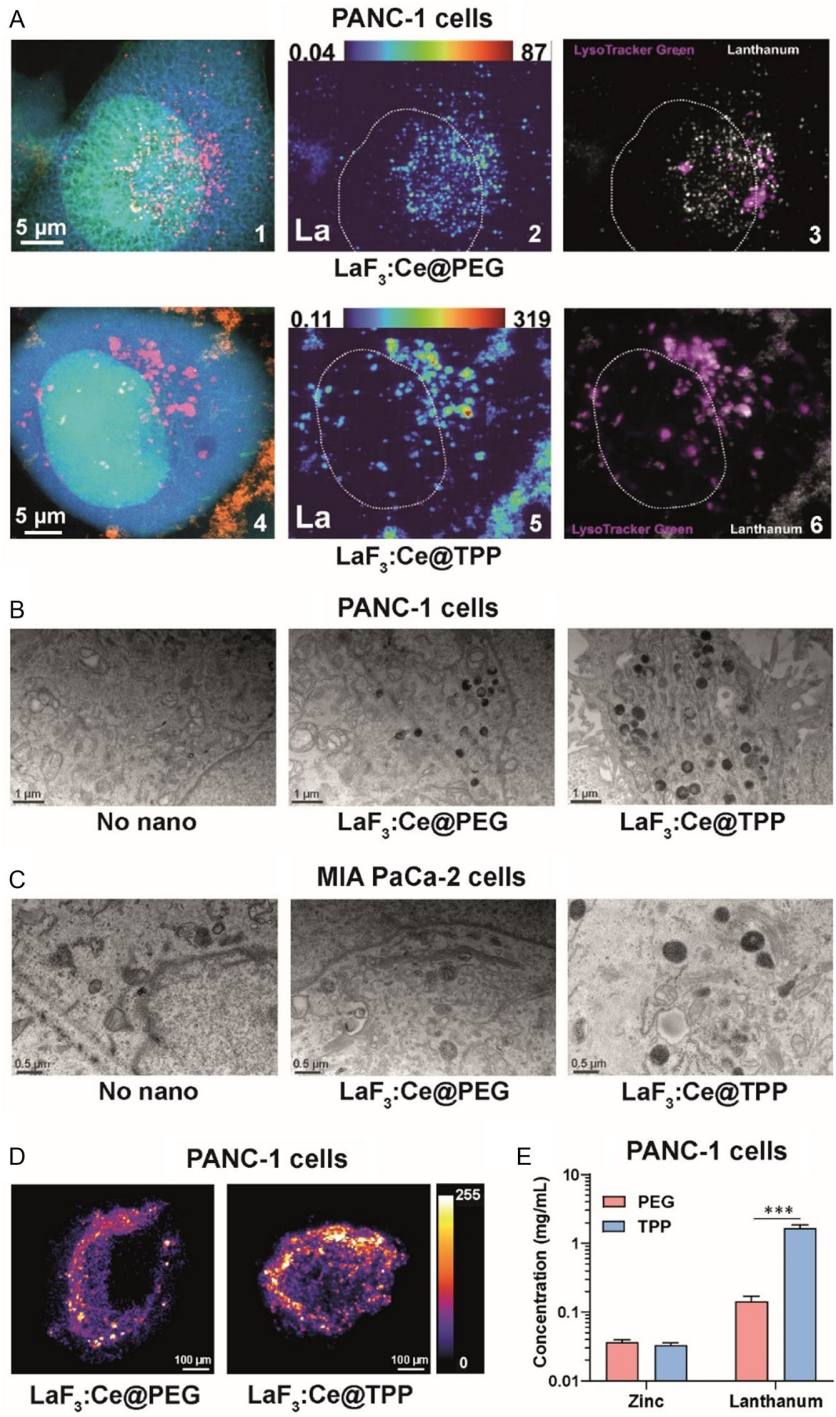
LaF_3_:Ce NPs internalize in lysosomes and diffuse throughout spheroids. A) XRF images of PANC‐1 cells previously incubated for 24 h with 0.1 mg mL^−1^ PEG‐ (1, 2, 3) or TPP‐ (4, 5, 6) coated NPs (scale = 5 μm): 1,4) zinc (nucleus, green), potassium (cytoplasm, blue), and lanthanum (NPs, red); 2,5) lanthanum; 3,6) lysosomes (lysotracker green, purple) and lanthanum (NPs, white). B,C) TEM images of PANC‐1 (B, scale = 1 μm) and MIA PaCa‐2 cells (C, scale = 0.5 μm) incubated for 24 h with PBS or 0.1 mg mL^−1^ NPs. D) CT images of XRF emission of lanthanum in PANC‐1 spheroids incubated for 24 h with 0.1 mg mL^−1^ NPs (scale = 100 μm). E) Zinc (cell) and lanthanum concentrations quantified using the XRF microtomography images.
